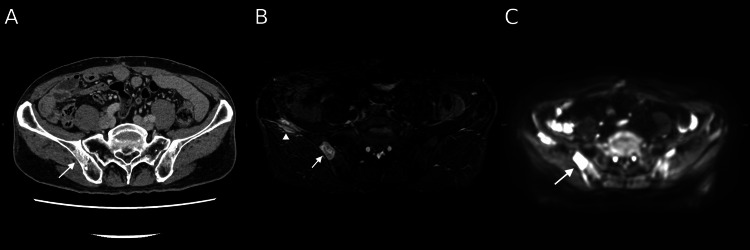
Multimodality imaging of the right iliac bone lesions Computed tomography exhibits ill-defined areas of increased medullary attenuation (A). Magnetic resonance imaging demonstrates slightly heterogeneous hyperintensity on short tau inversion recovery (STIR) sequence (B) and hyperintensity on diffusion-weighted imaging (C), with an apparent diffusion coefficient of approximately 1.2 × 10⁻³ mm²/s. One lesion extends into adjacent soft tissue, with preservation of the cortical bone (arrowhead). Percutaneous biopsy of one lesion (arrow) confirmed inflammatory myofibroblastic tumor.
